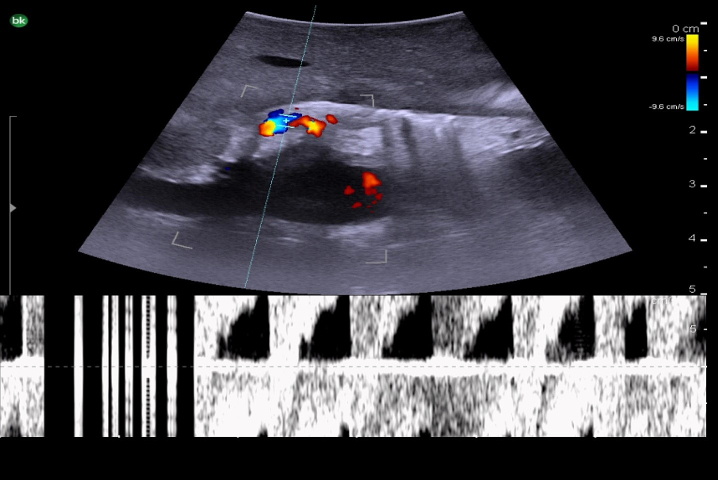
Intraoperative Doppler ultrasound of hepatic arterial flow. Note that there is no flow in the adjacent bile duct.
